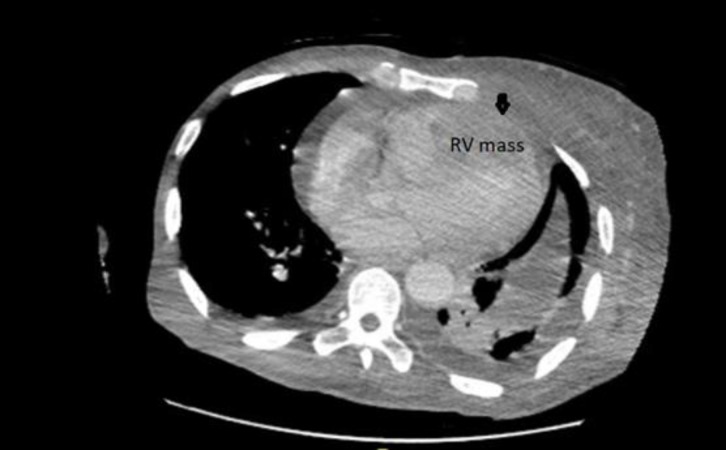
Cross sectional view of CT thorax showing a large right ventricular mass. Arrow: Deformation of right ventricular free wall suggesting infiltration of right ventricular mass into the right ventricular wall myocardium
